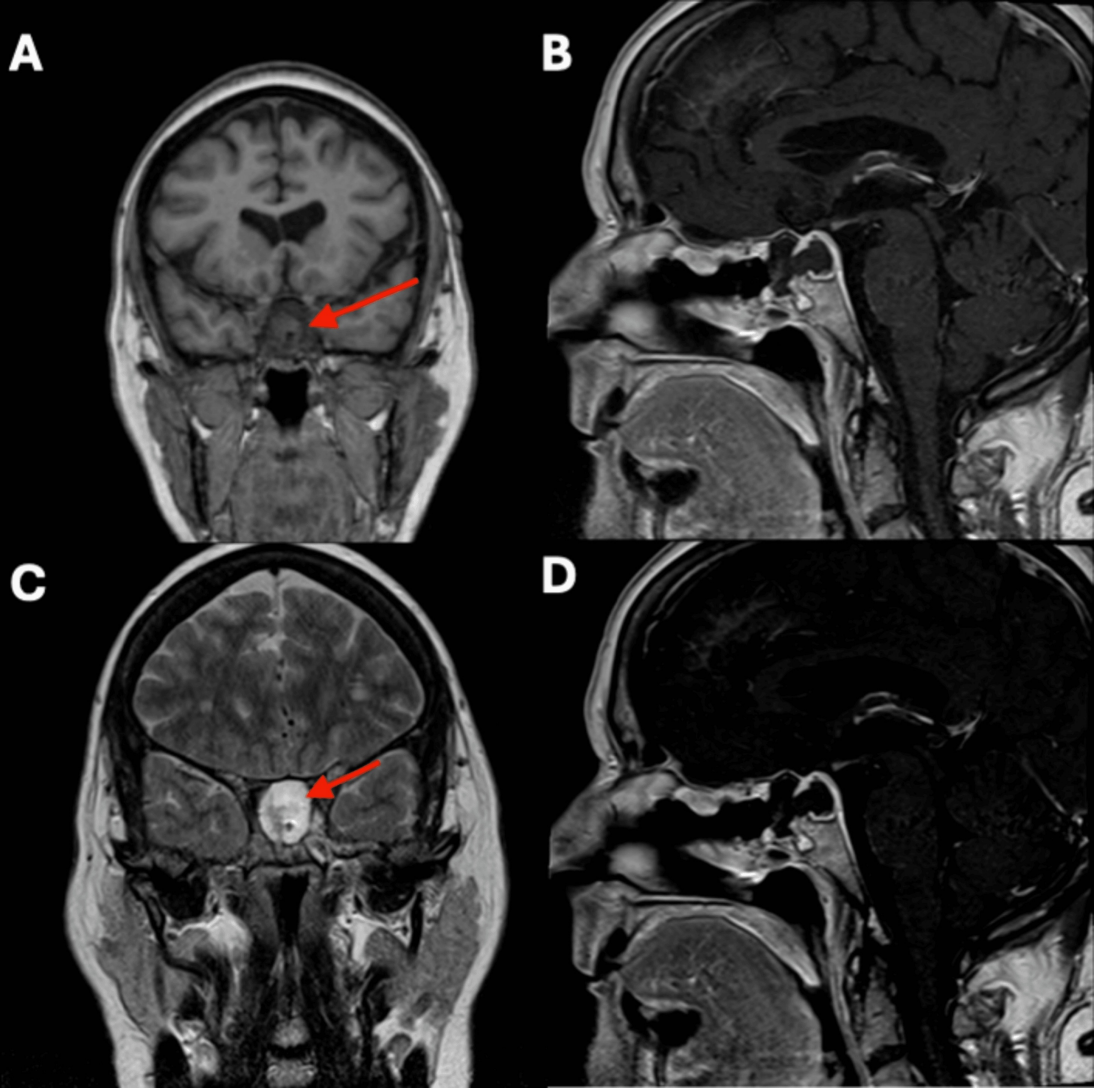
Coronal (A) and sagittal (B) T1-weighted MRIs and coronal (C) and sagittal (D) T2-weighted MRIs one month post-transsphenoidal resection of the pituitary neuroendocrine tumor The red arrow indicates the fluid-filled sella turcica and suprasellar cistern, measuring 1.8 cm cephalocaudally, without a solid mass.
